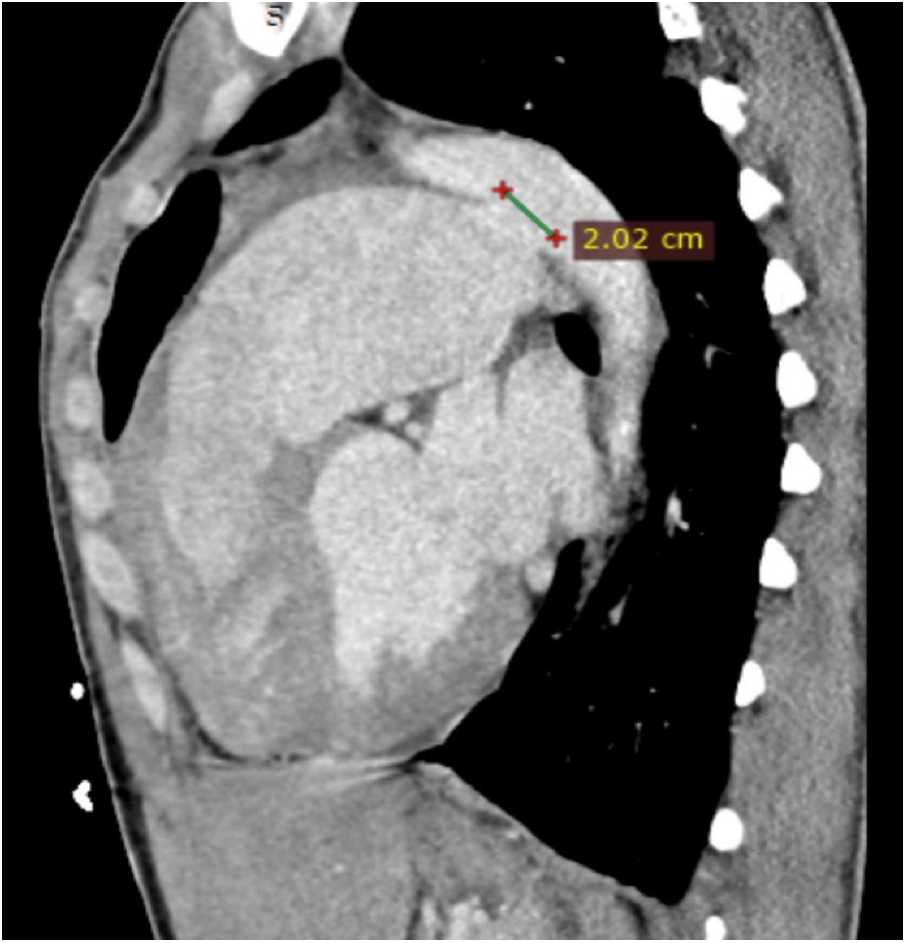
Cardiac CT in sagittal view shows a large PDA (20.2 mm) with a dilated pulmonary artery. CT, computed tomography; PDA, patent ductus arteriosus.
